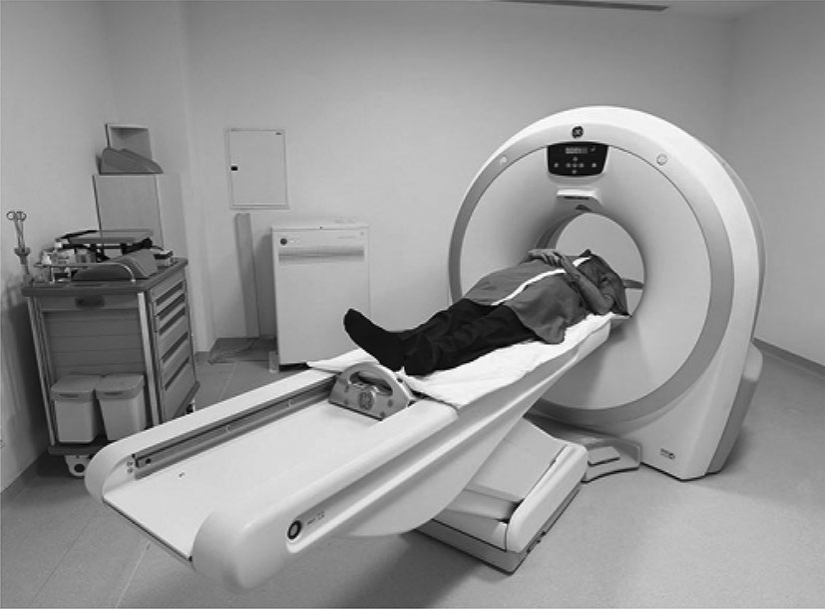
Example of magnetic resonance imaging (MRI) scanner setup in an authorized private medical hospital producing high-quality images of human brain. The motorized bed is moved inside the oval shaped scanner, and the head is scanned
